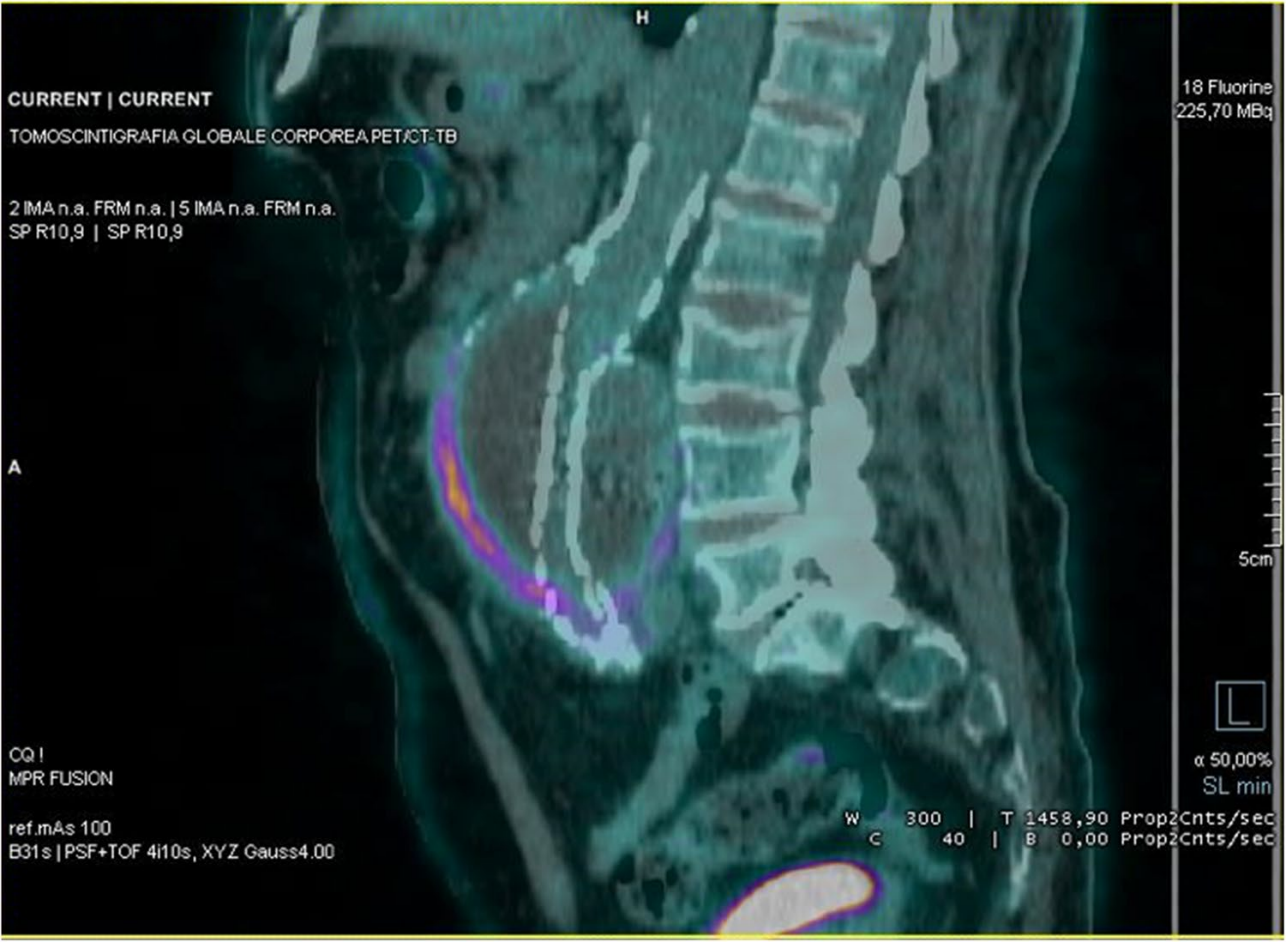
Sagittal view of [^18^F]FDG PET/CT shows mild and homogeneous [^18^F]FDG uptake around the aneurysmatic sac without endograft involvement
